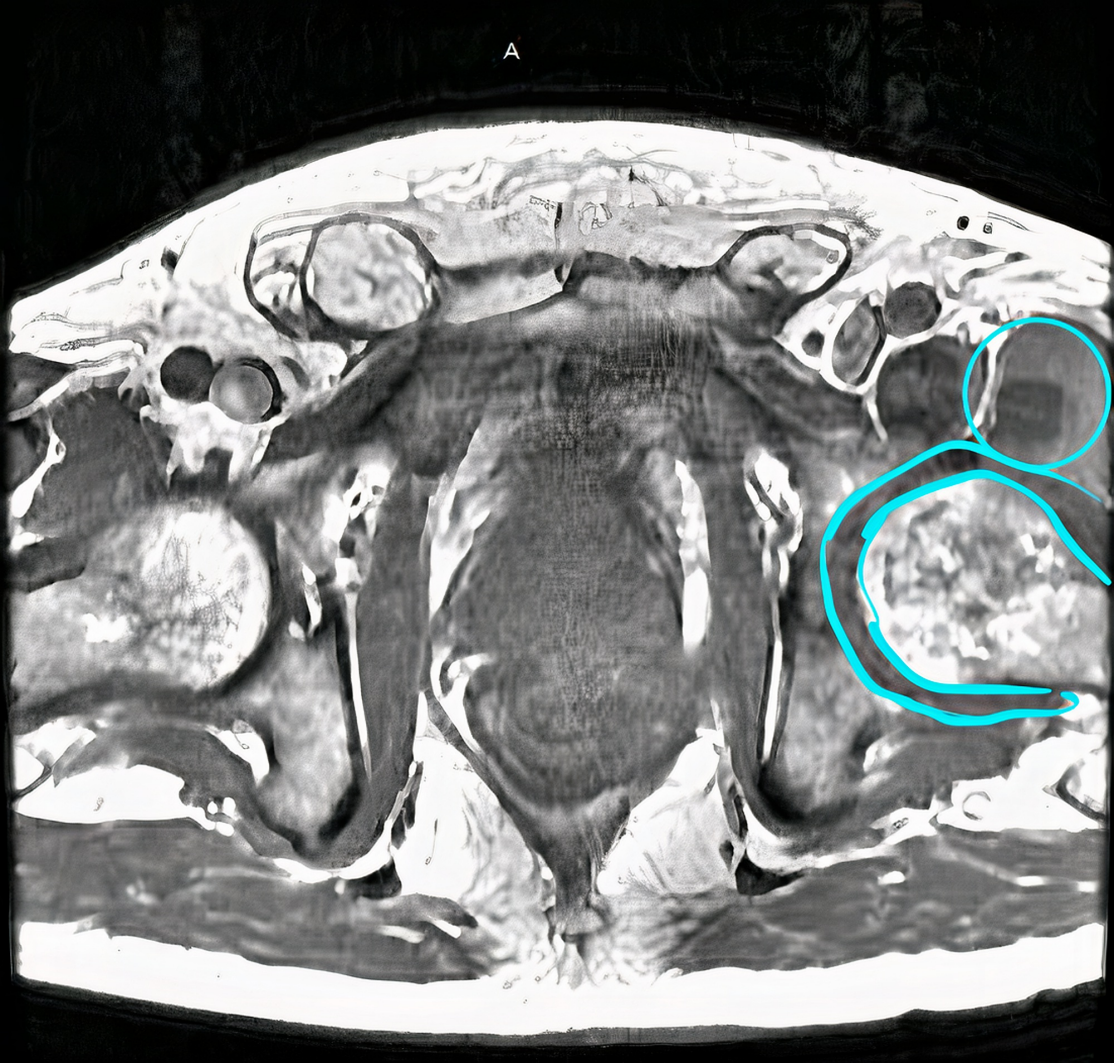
MRI abdomen/pelvis showing left anterior abscesses (blue circle) and joint capsule inflammation (blue outline).
